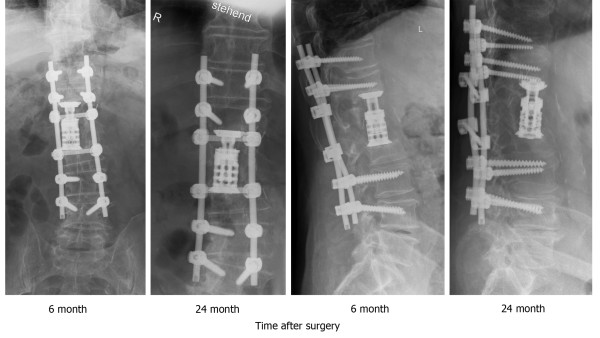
Anterior posterior (AP) and lateral plain thoracolumbar radiographs six and 24 months after reconstruction and spondylodesis (T11 - L4). We performed the transpedicular fixation with a dual rod system and vertebral replacement of the L1 vertebra using an expandable cage. Reconstruction is stable on both AP and lateral views at six months. Follow-up radiographs at 24 months show no signs of pseudarthrosis or infection.
